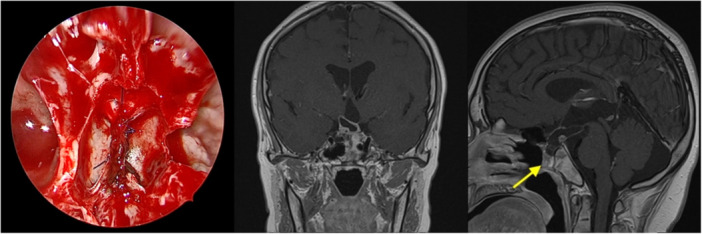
Stalk of the ligated herniated sac and postoperative magnetic resonance imaging images indicated the disappearance of the mass in the posterior nasal cavity. The arrow indicates the nasoseptal flap.
